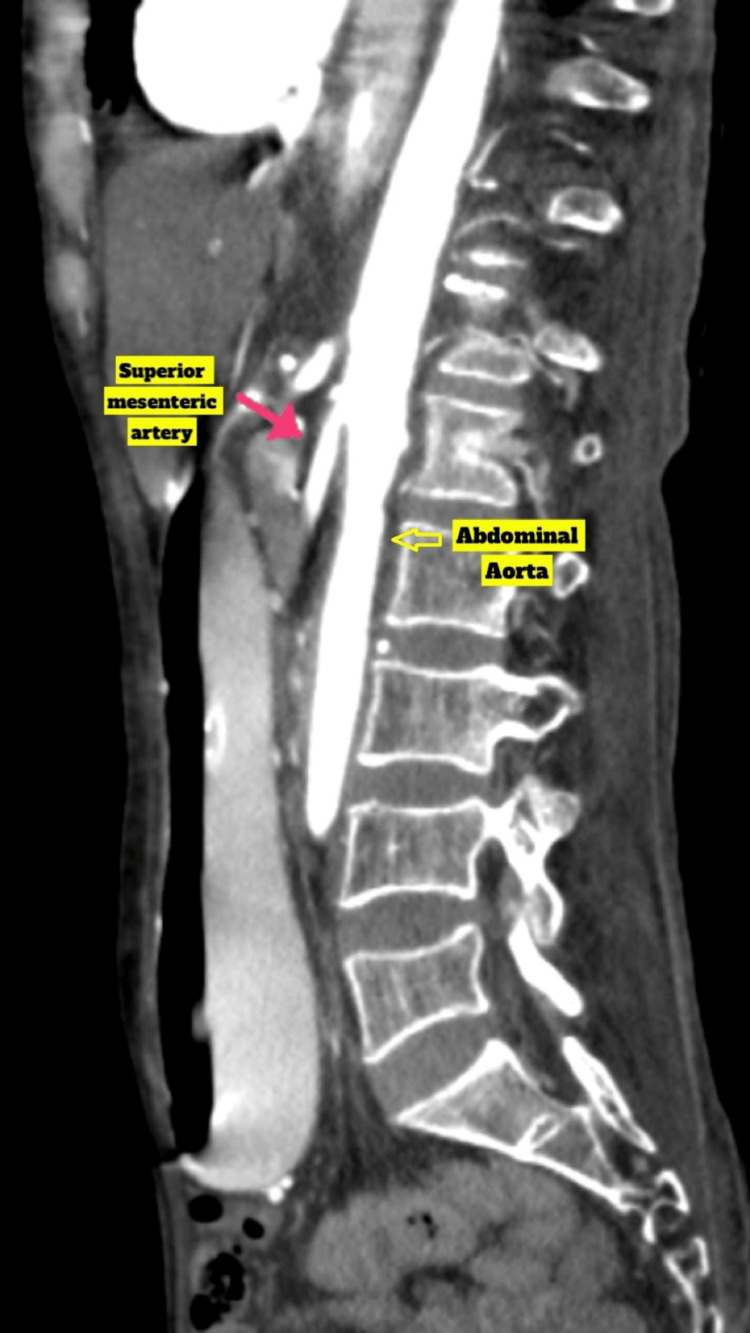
CT with angiography revealed decreased aorto-mesenteric angle and distance
